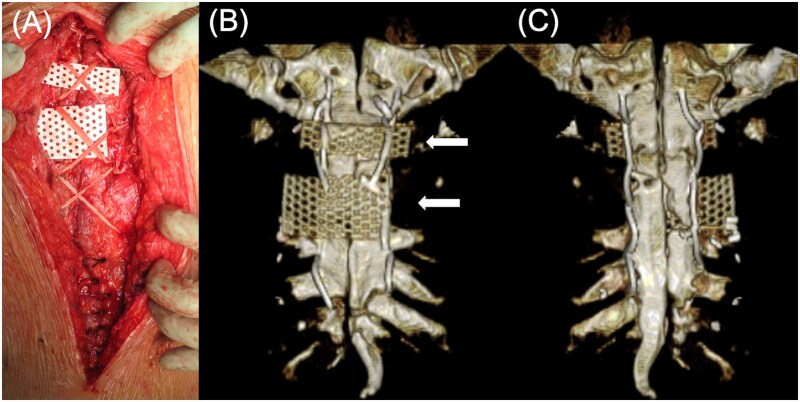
Intraoperative and Postoperative 3 D-CT Findings After Definitive Sternal Reclosure Using Ultra-High Molecular Weight Polyethylene Tape. (A) Intraoperative view showing fractured sternal segments approximated in a figure-of-eight configuration with mesh plates to prevent bone cut-through. (B) Anterior and (C) posterior views of postoperative 3 D-CT demonstrating satisfactory sternal approximation and alignment. White arrows indicate mesh plates.
